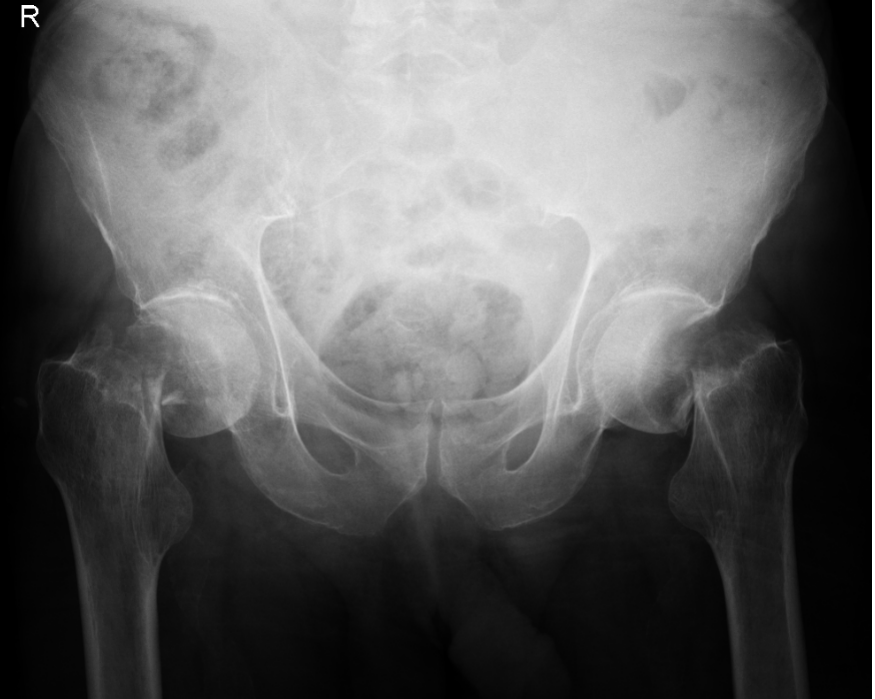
Anterioposterior radiograph of the pelvis showing bilateral completely displaced intracapsular fractures of the neck of femur.
